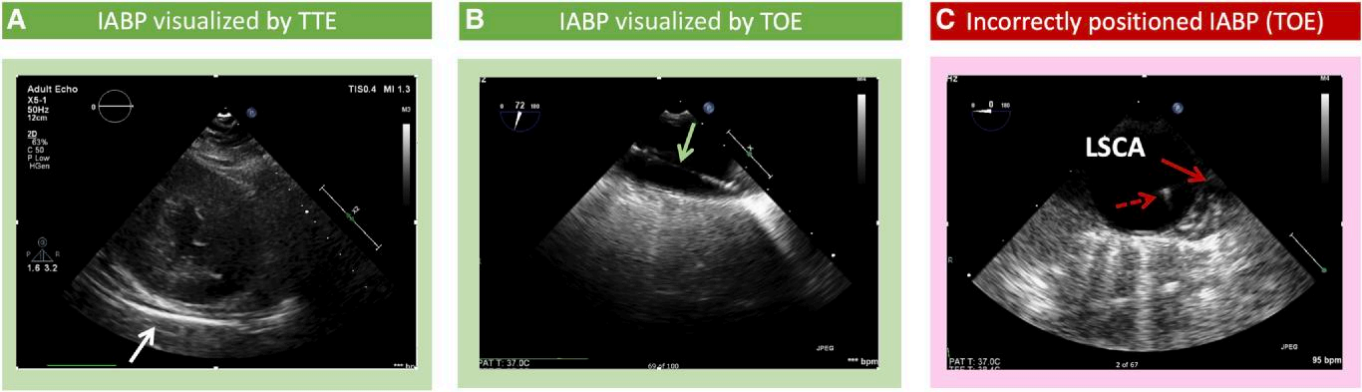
Echocardiography in IABP. (*A*) Left parasternal short-axis view showing an IABP in the descending thoracic aorta (white arrow). (*B*) TOE descending aorta long-axis view (70°) showing the IABP in its long axis (green arrow). (*C*) TOE descending aorta short-axis view (0°) showing incorrectly positioned IABP with the tip (dashed red arrow) visualized at the level of LSCA (solid red arrow).
